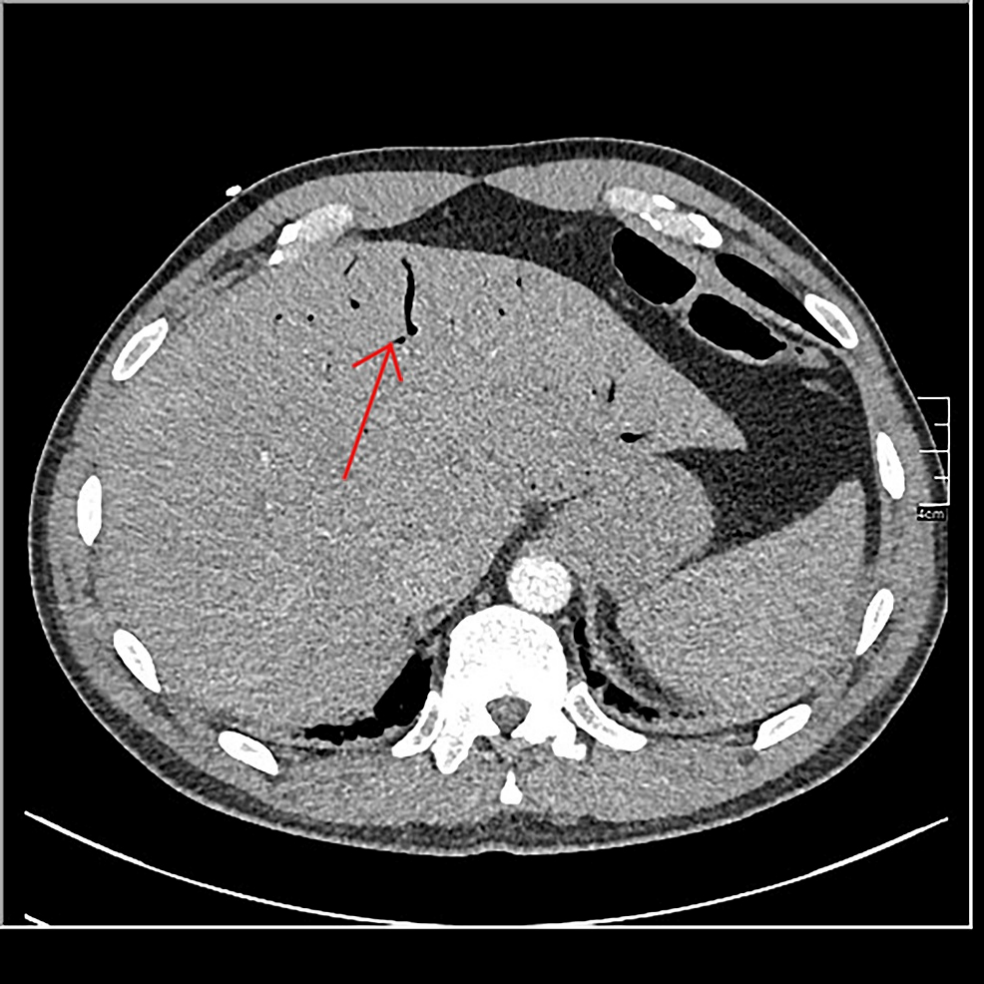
Abdominal CT scan of the liver showing air within the portal radicles. CT, computed tomography
